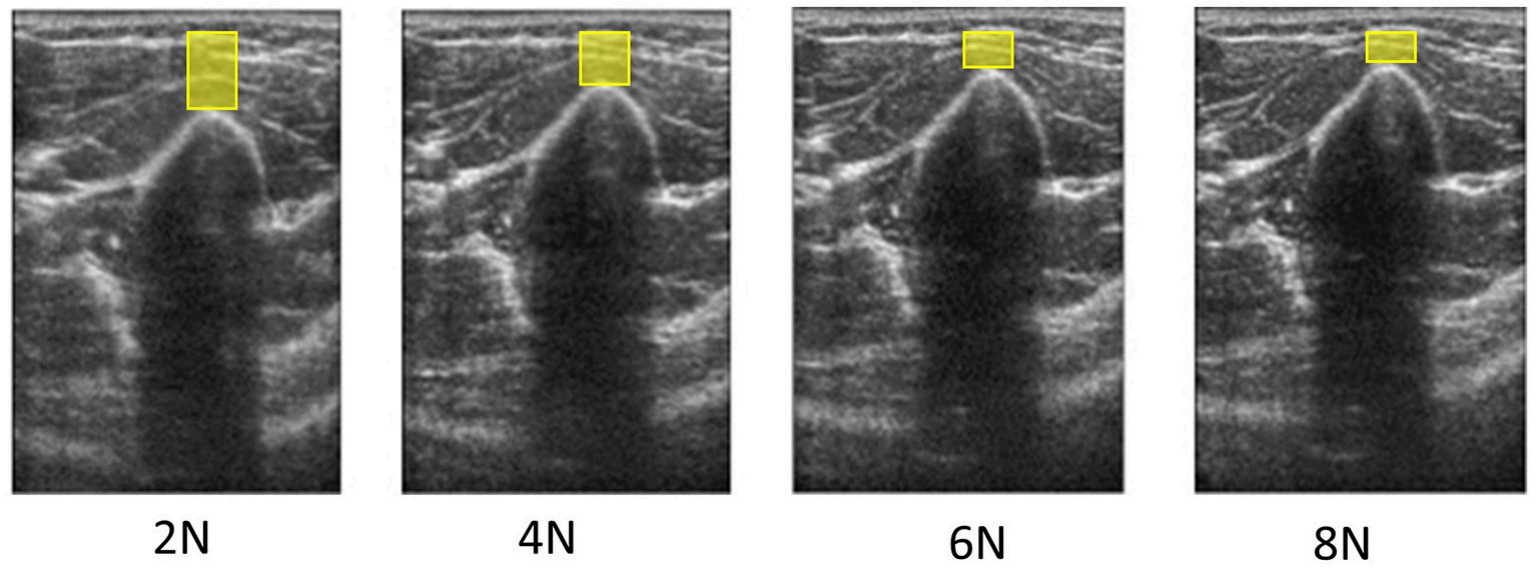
Ultrasound images of the biceps from a healthy subject at four different forces. Variation in the muscle thickness (denoted by the height of the yellow boxes), based on the measurement from the bone to the subcutaneous fat-muscle separation layer, is highly dependent on the examiner-generated force during scanning.
